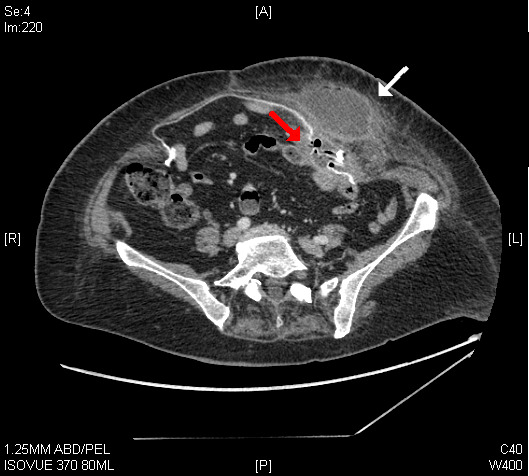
Computed tomography showing extraperitoneal (white arrow) and intraperitoneal (red arrow) abscesses associated with mesh and small intestine.
